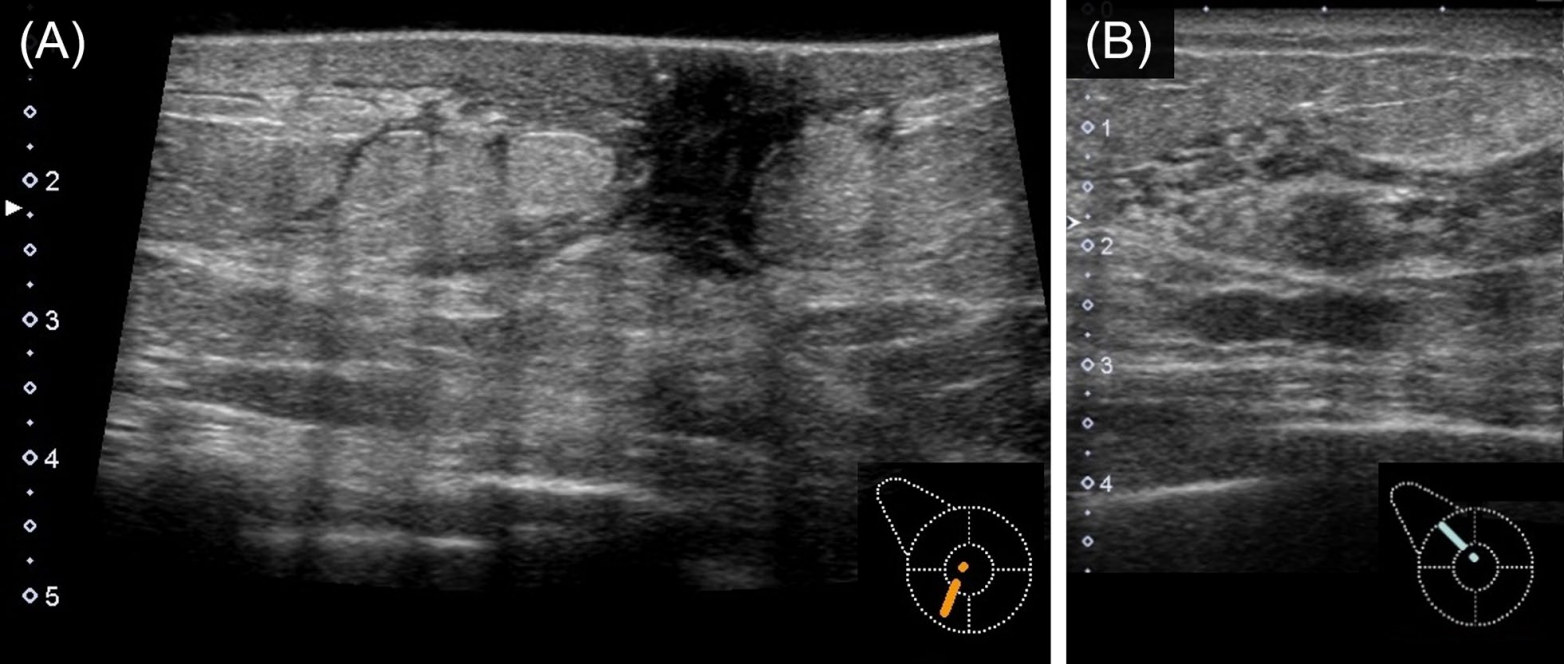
Ultrasonography of the right breast. A Thickening of the skin was observed at the lower lateral region. An increase in the echogenic level and thickening of the entire mammary gland was also seen compared to the contralateral breast. B A hypoechoic area with indistinct boundaries was seen within the mammary gland in the upper lateral area. It was accompanied by a slightly increased echogenicity of the surrounding fatty tissue
